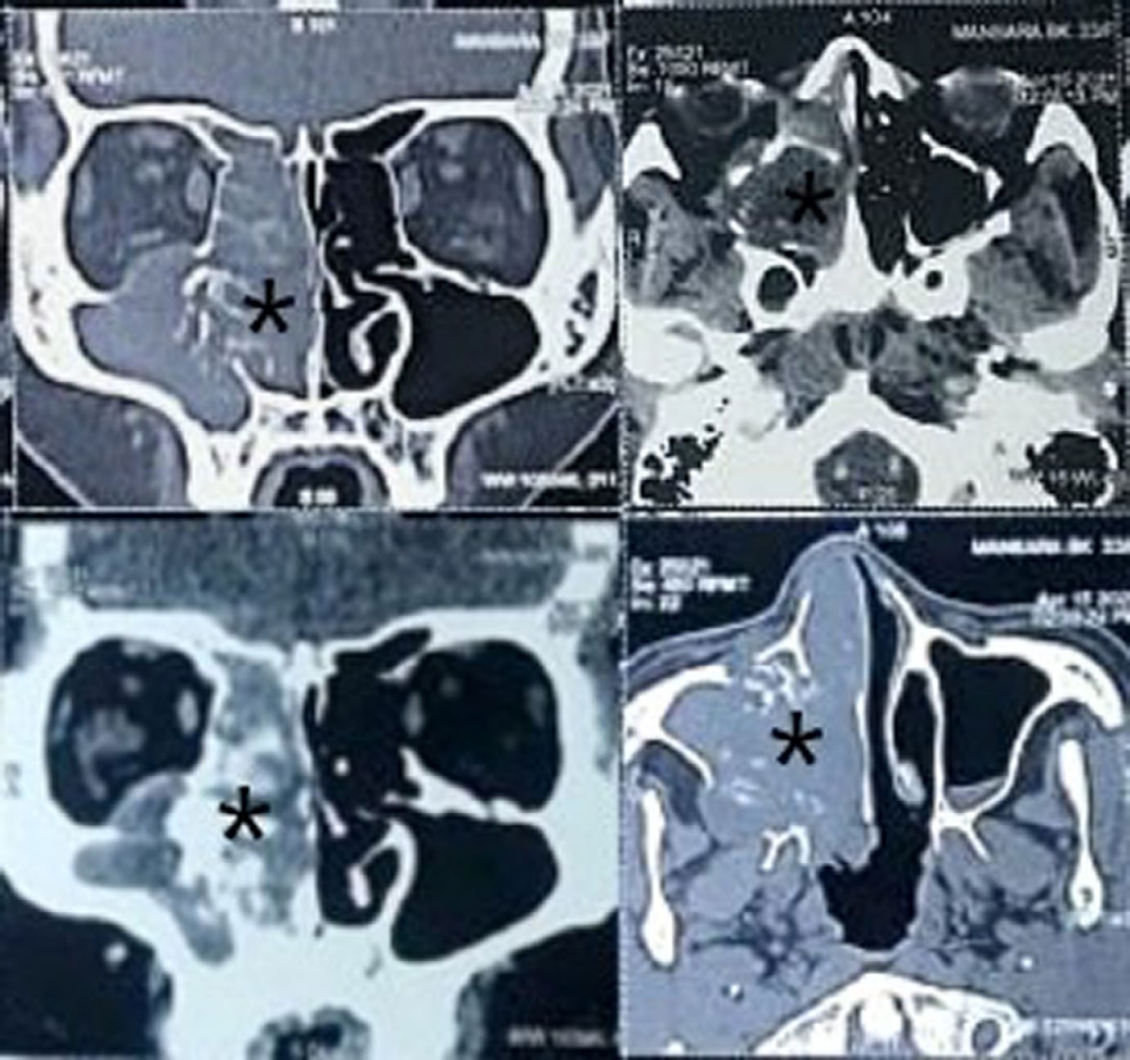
CT nose and PNS demonstrating soft tissue opacification in the right maxillary sinus, ethmoid and frontal sinus along with the erosion of surrounding bony structures
